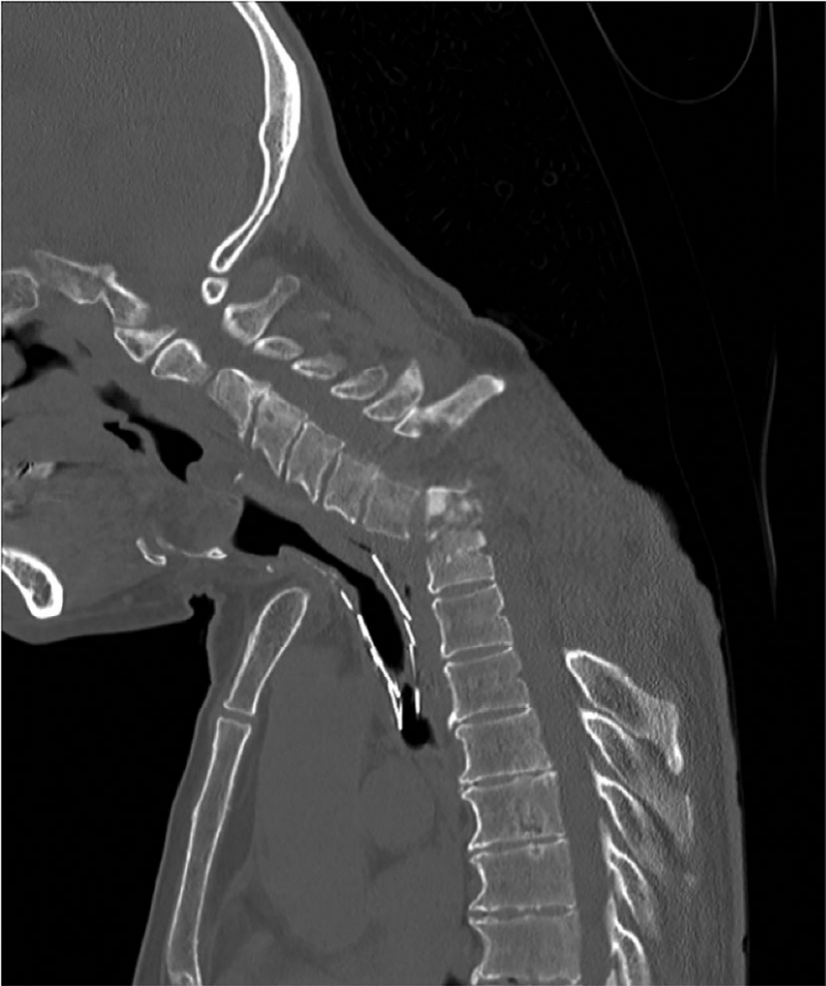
Computed tomography (CT) findings obtained on symptom recurrence. CTscan showed osteolytic destruction and kyphotic deformity at the T1–T2 level. The infection was uncontrolled and had spread to the surrounding areas.
